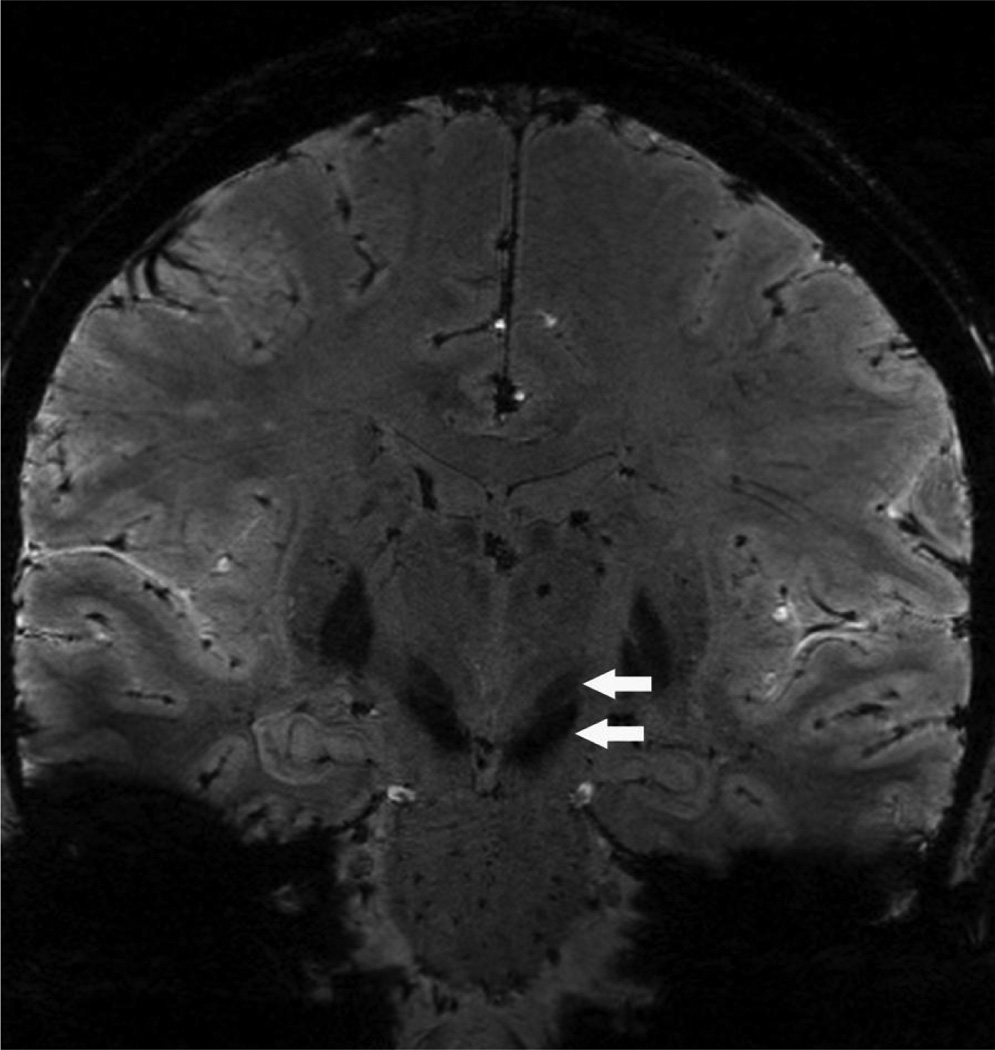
Susceptibility weighted imaging (SWI) acquired at a high-field (7T) MRI. Image resolutions 0.4 × 0.4 × 0.8 mm^3^. Coronal view shows a clear delineation between the subthalamic nucleus (STN) (higher arrow) and the substantia nigra (lower arrow). Images courtesy of Dr. Noam Harel, University of Minnesota.
